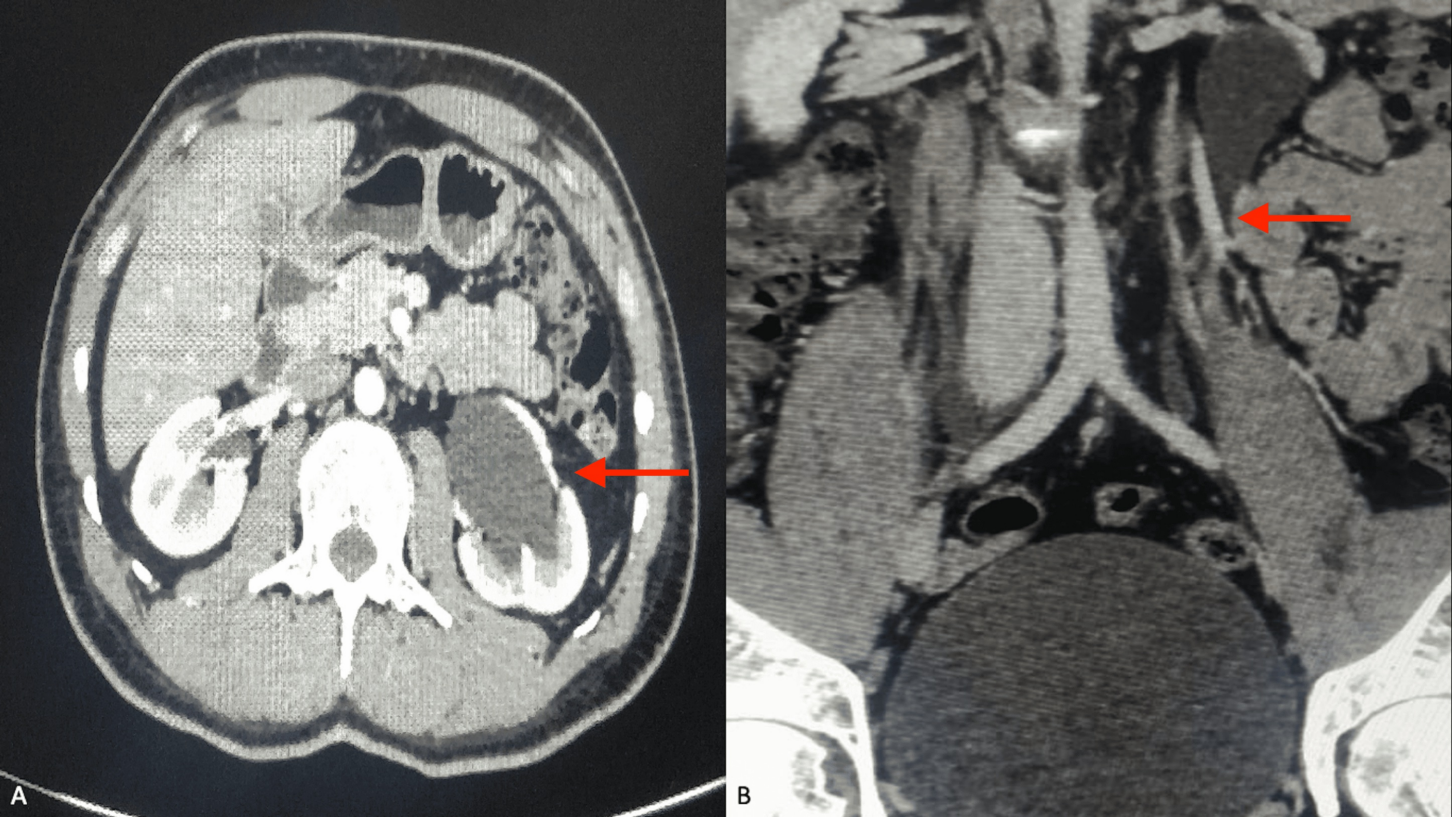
Multidetector CT scan showing compression of the left ureter by the crossing IMV (B), causing upstream hydroureteronephrosis (A) IMV: inferior mesenteric vein
